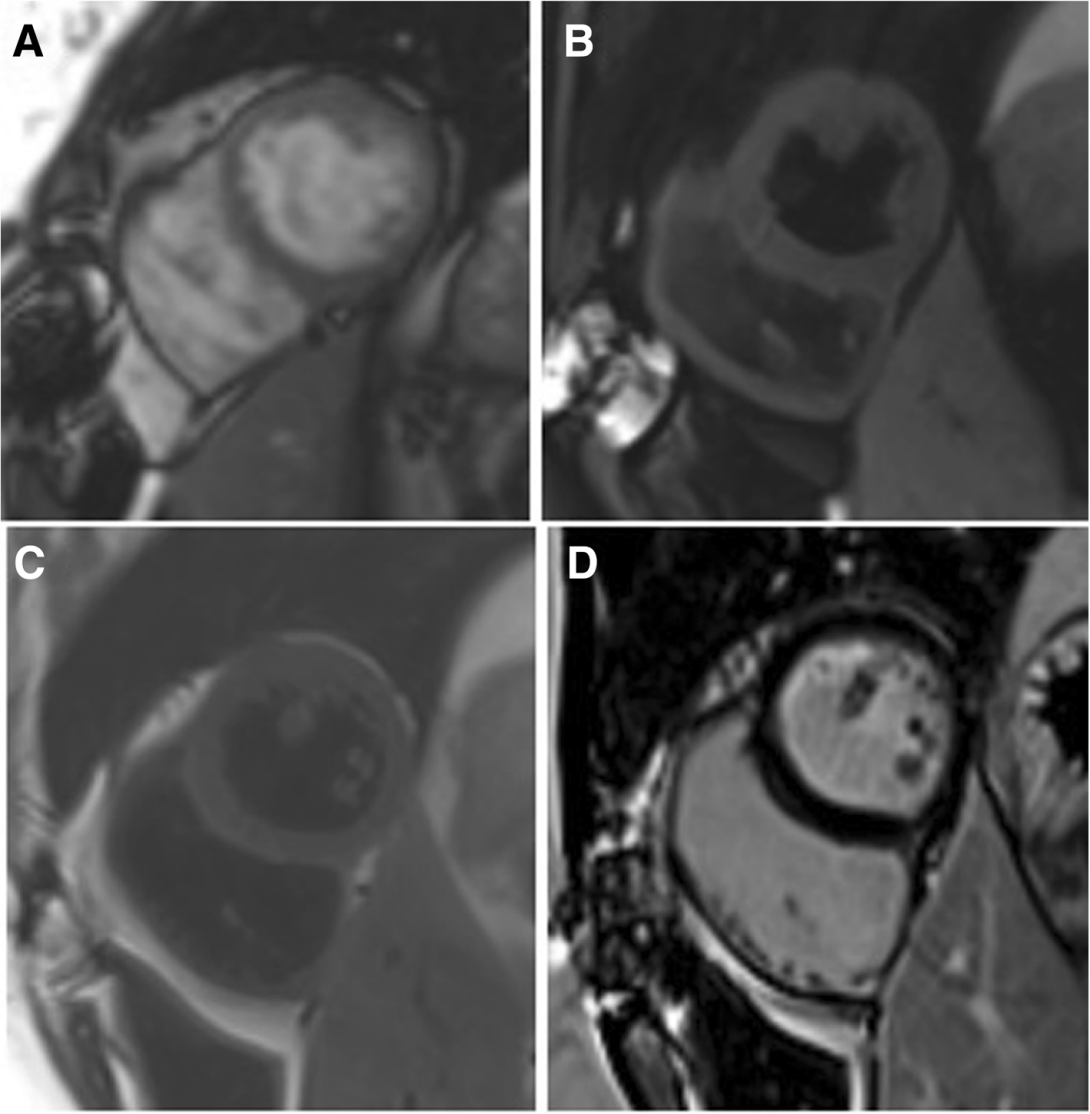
CMR after 26 months from the diagnosis. Still in complete response in cine (a), T1-weighted (b), T2-weighted (c) and LGE (d) images
